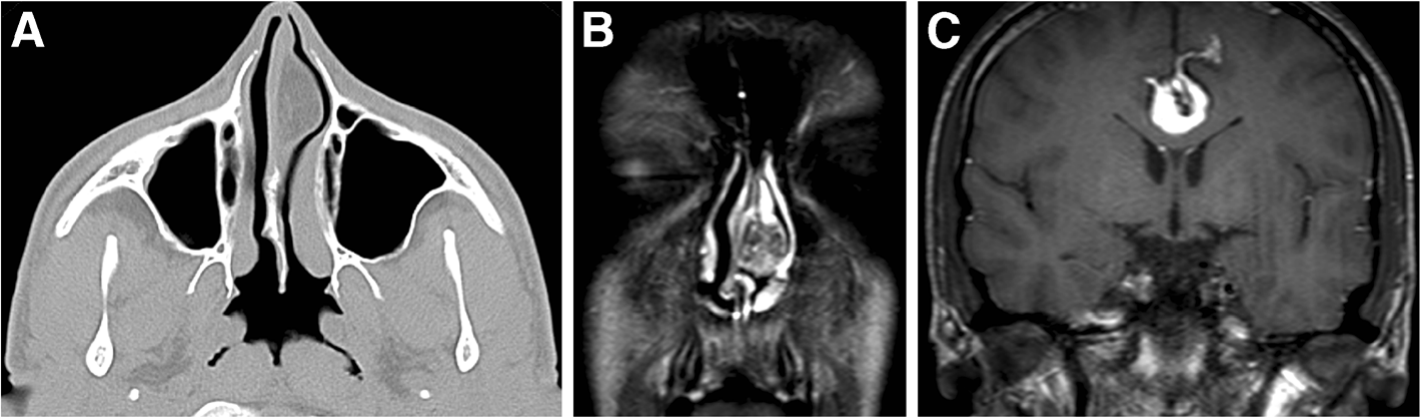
(A) Computed tomography showing a 25x12 mm low density mass in the nasal septum. (B) T2 weight magnetic resonance (MR) imaging presenting a high signal intensity 22x12 mm mass. (C) MRI showing a lipoma, 18 mm thick and 51 mm in length at the level of falx cerebri that pushed the corpus callosum and reaching the cingulate gyrus anteroposteriorly.
